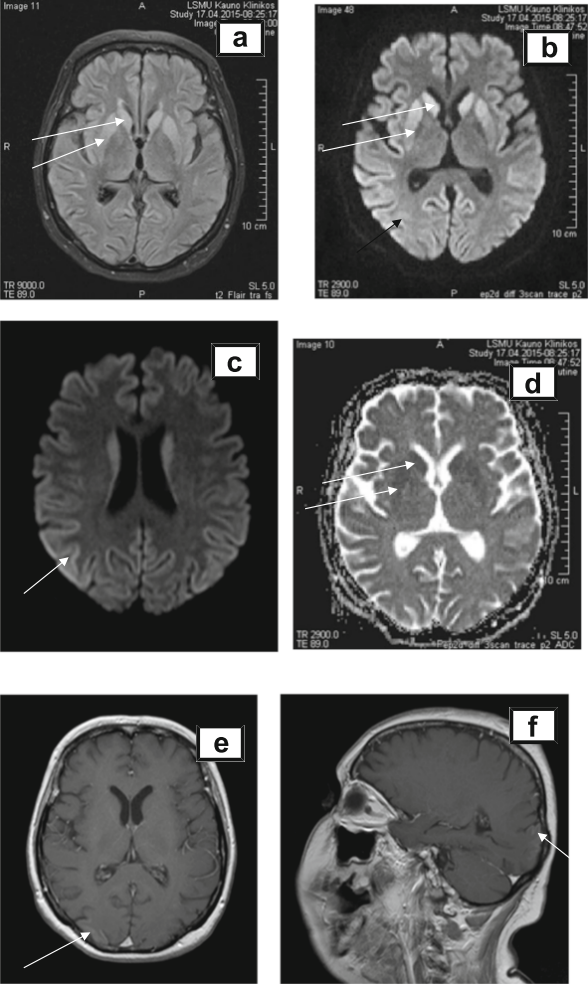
Magnetic resonance images at week 13. Legend: hyperintense lesions in the basal ganglia (head of *nucleus caudatus, nucleus lentiformis,* arrows) in T2W, DW sequences (a, b, c, d
